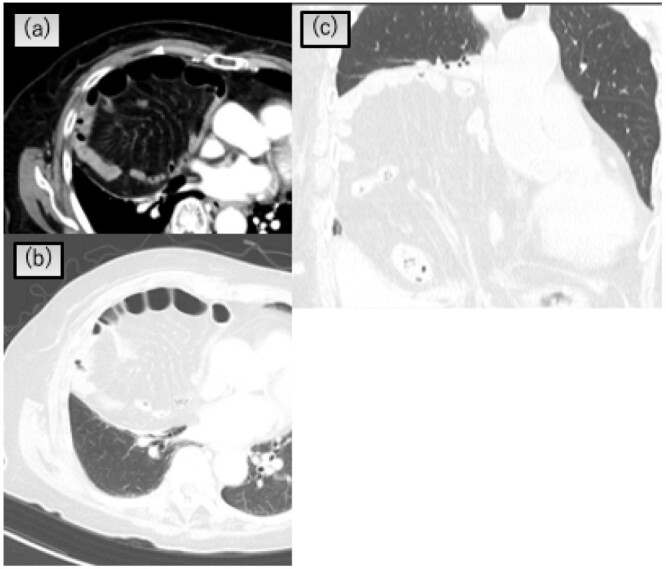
Chest CT scan showing that the transverse colon and greater omentum in the right pleural cavity and atelectasis of the right lower lobe. (a) and (b) Axial view, (c) coronal view.
